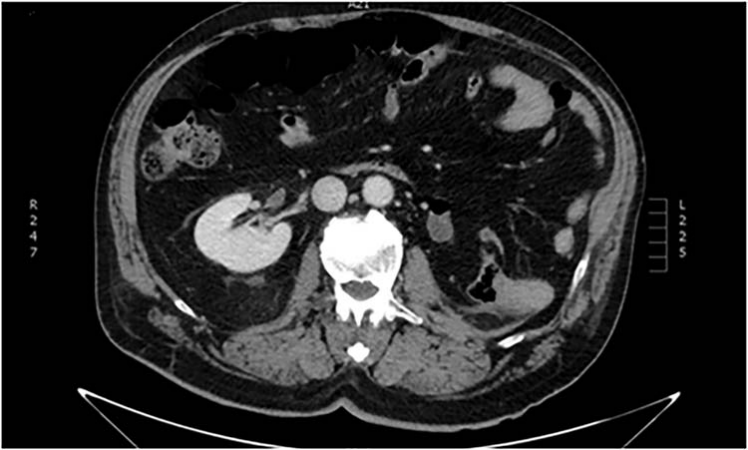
Abdominal CT imaging. Absence of left kidney from its usual anatomic position.
